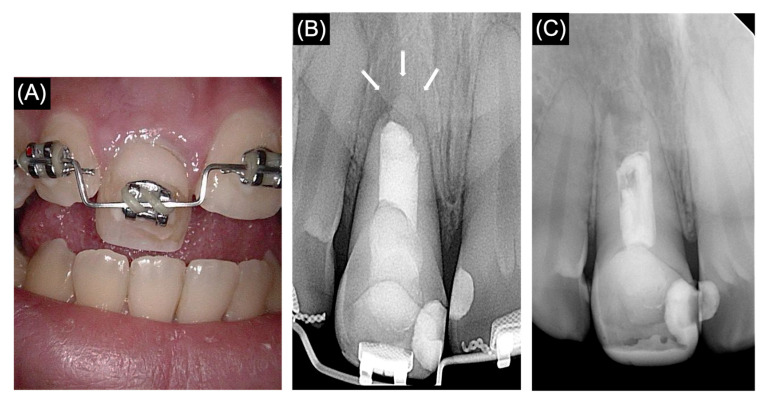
(A) Clinical photograph of tooth number 11 at the two-year follow-up visit. (B) Two-year follow-up periapical radiograph of tooth number 11. (C) Preoperative periapical radiograph of tooth number 11.
